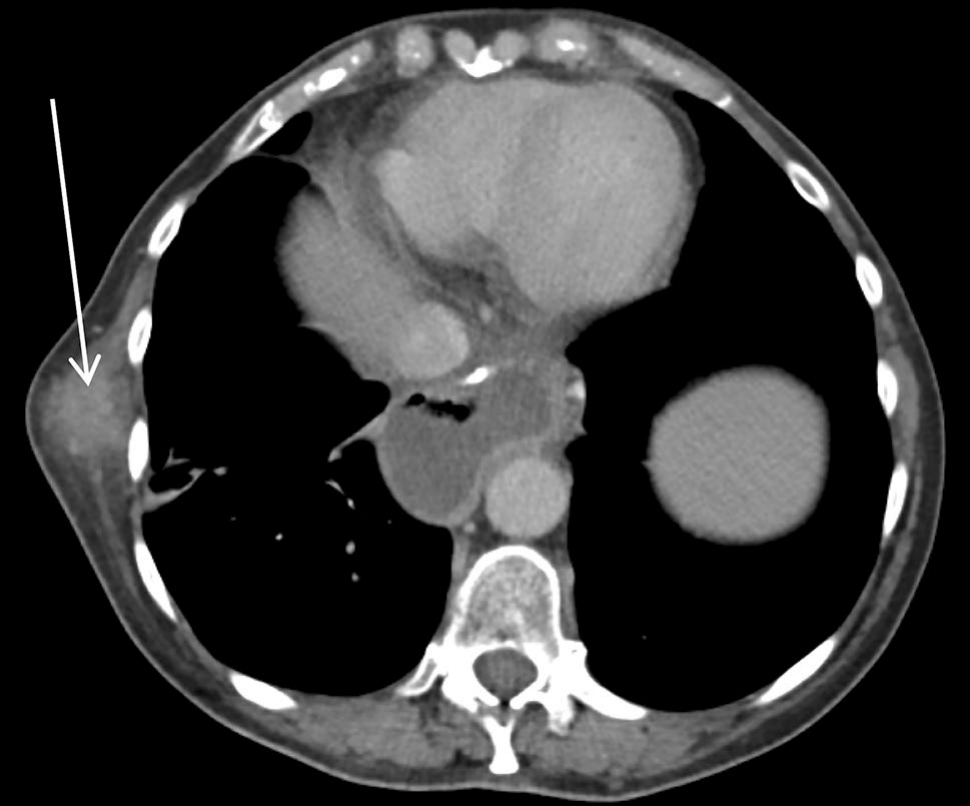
CT scan showing right lateral chest wall mass
